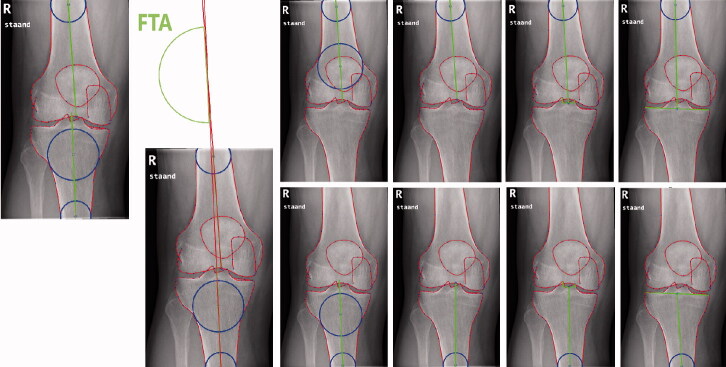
Measurement of the femoro-tibial angle (FTA) on standard knee radiographs. Tib4 Fem4 Tib3 Fem3 Tib2 Fem2 Tib1 Fem1
